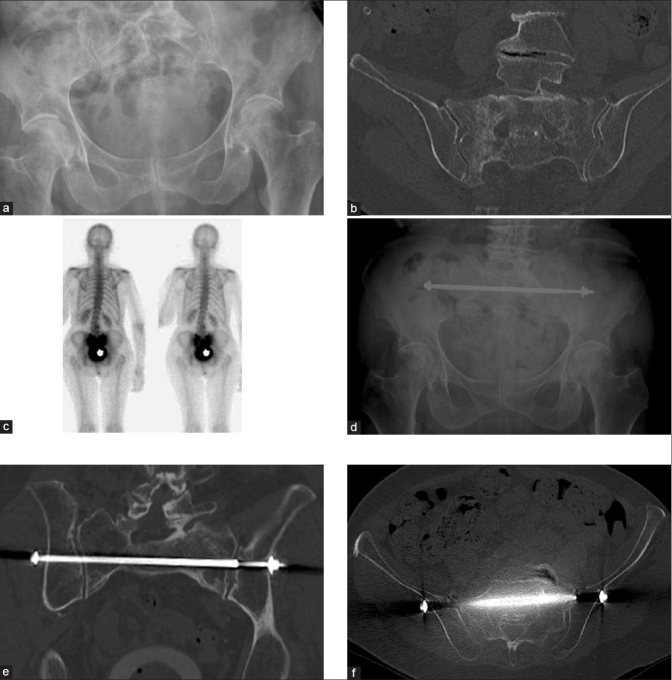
76 years old women with complaint of incapacitating low back pain radiating to the left lower extremity (S1 topography). Plain radiographs were unhelpful to make a diagnosis (a). An axial CT scan with coronal and sagittal reconstructions showed bilateral insufficiency fractures of the sacrum (b). A bone scan using 99mTc-MDP tracer showed an increased uptake at the level of both sacro-iliac joints (c). A TISIB-procedure has been performed since the insufficiency fractures occurred bilaterally (d). A postoperative CT scan with axial reconstructions showed a well-positioned bar at the level of S1 (e). A postoperative CT scan with coronal reconstructions showed a well-positioned bar at the level of S1 (f).
